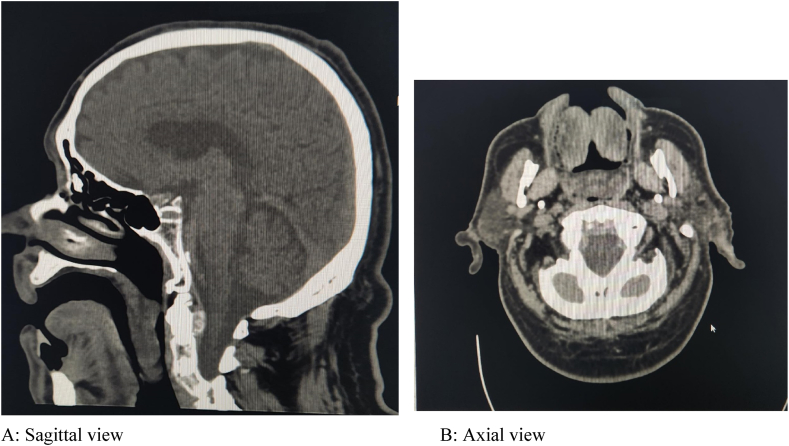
Brain CT scan, A: Sagittal view, B: Axial view: A pedunculated soft tissue mass measuring approximately 5 × 2 cm in the oropharynx, attached to the soft palate.
